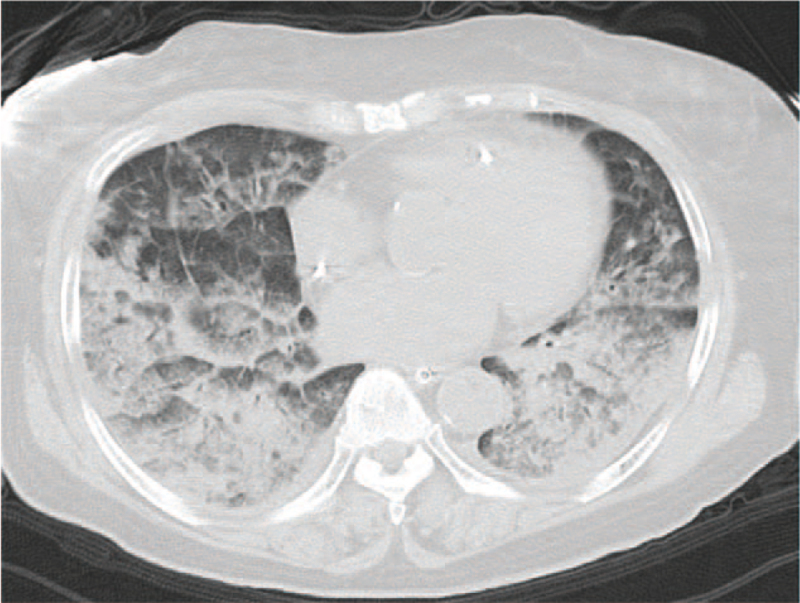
Computed tomography of the lungs. Chest computed tomography showed bilateral diffuse alveolar infiltrates.
